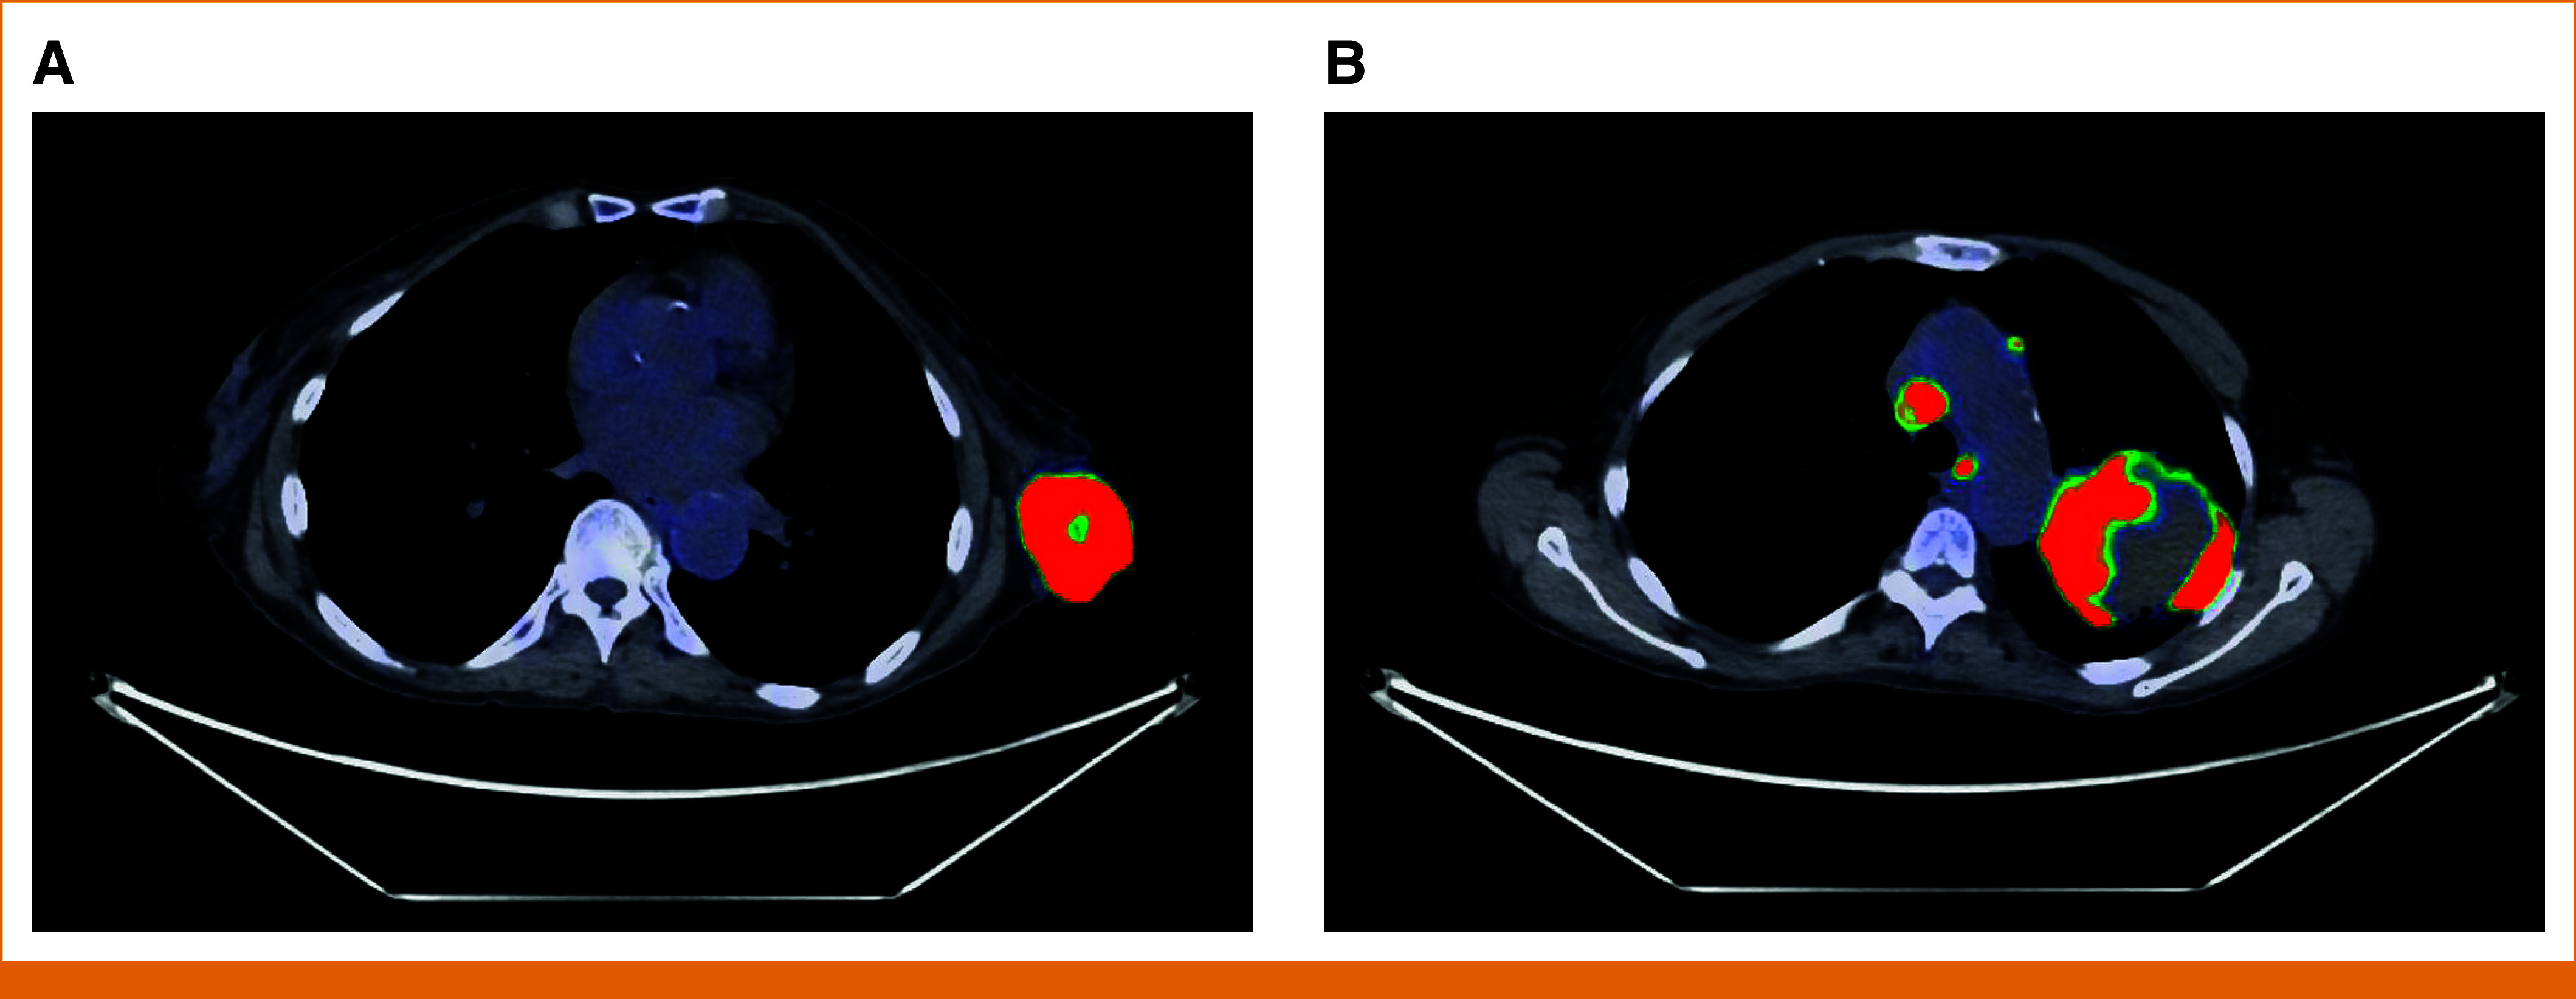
(A) Intensely hypermetabolic left breast mass approximately 4.5 cm in diameter, maximum SUV 26.4, on FDG PET-CT. (B) Greater than 7 cm intensely hypermetabolic left lung consolidation, maximum SUV 33.3. Hypermetabolic right supraclavicular, mediastinal, and right axillary/subpectoral lymphadenopathy was also noted on PET-CT, with a maximum SUV of 28.9 involving right supraclavicular lymphadenopathy. Additional hypermetabolic subcentimeter pulmonary nodules, the largest subpleural measuring 0.7 cm in the right lower lobe with a maximum SUV of 6, and 0.5-cm pericardial nodule with mild hypermetabolism, with a maximum SUV of 3.5, were also visualized. FDG PET-CT, fluorodeoxyglucose positron emission tomography-computed tomography; SUV, standardized uptake value.
